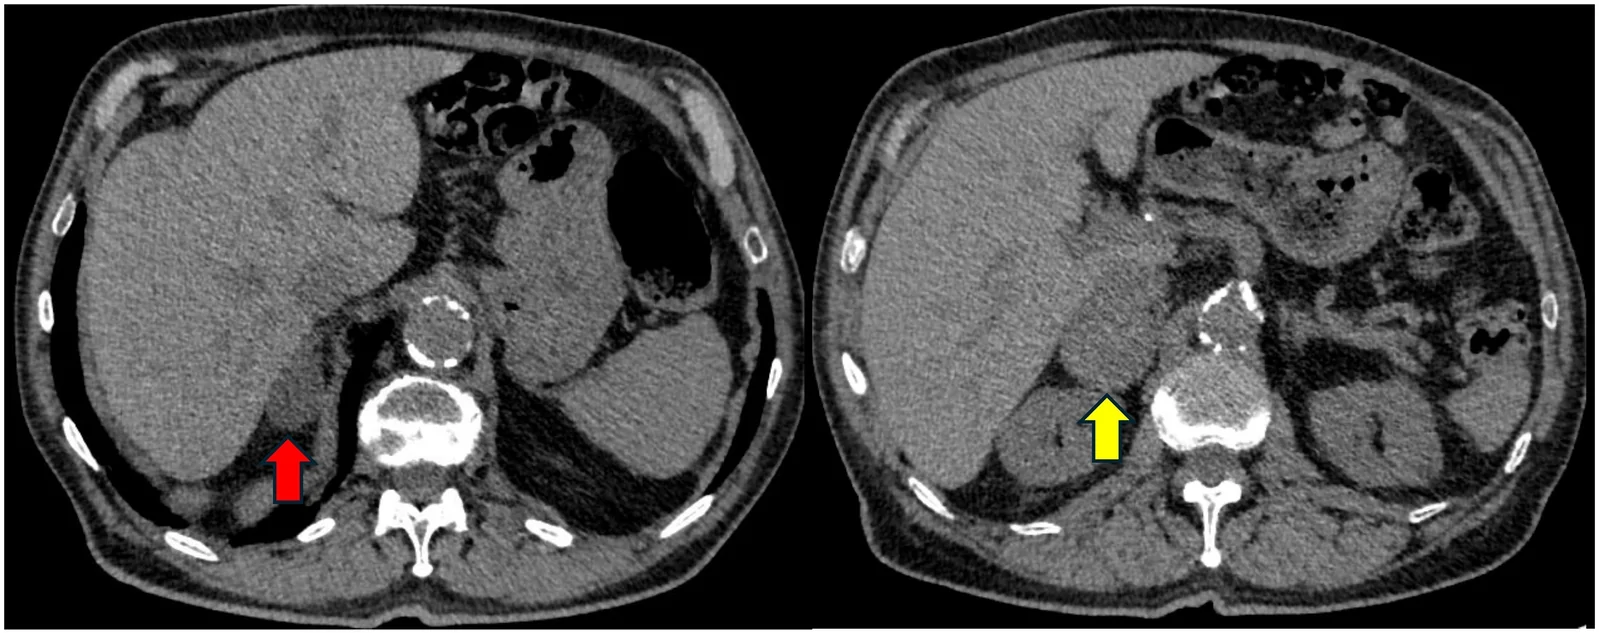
Unenhanced CT of right adrenal gland lesions. Unenhanced abdominal CT scan showing 2 nodular lesions in the right adrenal gland: a 31 × 20 mm low-density lesion (HU close to 0), consistent with an adenoma (left arrow), and a 44 × 38 mm high-density lesion (30-40 HU) located at the inferior pole of the gland (right arrow).
